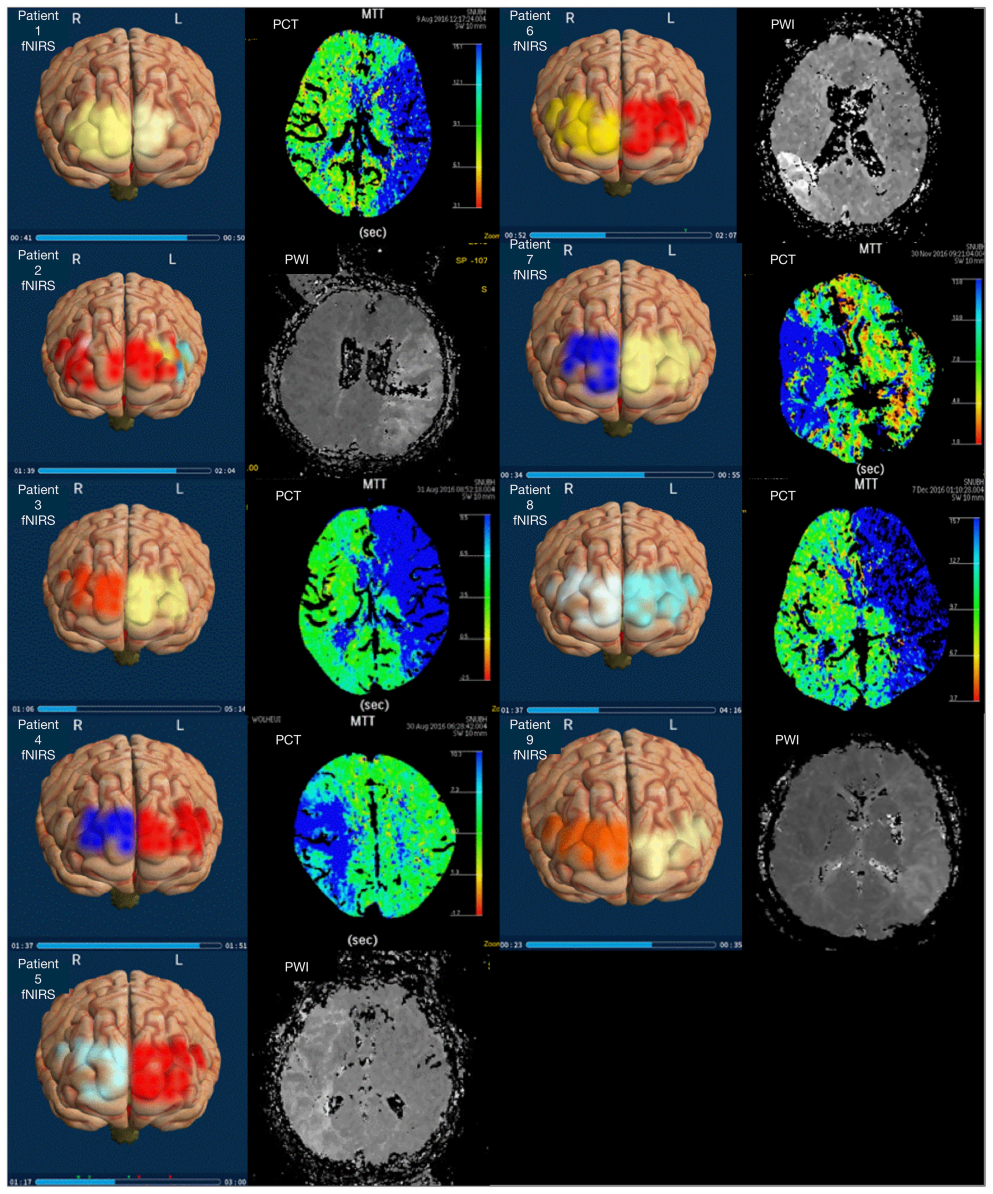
Images from fNIRS vs. PCT or PWI. This figure shows the comparison of fNIRS images and PCT or PWI images from MCA infarction patients. Patient 1 showed poor or brighter yellow color of the left frontal lobe on the 3D display (left side) and a perfusion delay (blue color) in the left MCA territory on the MTT map (right side). Patient 2 showed asymmetric yellow and blue coloration of the left frontal lobe and a perfusion delay in the left MCA territory on the TTP map (white color). Patients 3, 4, 7, and 8 showed a perfusion delay on the MTT map with compatible asymmetric color mapping of the 3D frontal cortex. Patients 5, 6, and 9 a showed perfusion delay on the TTP map with asymmetric color mapping. The TTP map from PWI showed a perfusion delay in the whole left MCA territory with a multifocal, small-diffusion restricted lesion in the left MCA territory on diffusion-weighted imaging (DWI). The average right and left cerebral rSO2 values were 87.15 and 68.25%, respectively. No, number; R, right; L, left; MCA, middle cerebral artery; MTT, mean transit time; sec, second; PCT, perfusion computed tomography; PWI, perfusion-weighted magnetic resonance image.
